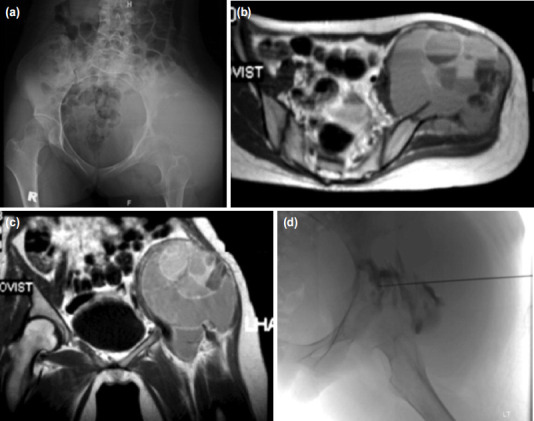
(a) Plain radiograph of pelvis showing a lytic lesion at left iliac region, without involvement of the left acetabulum and a narrow zone of transition with soft tissue shadow extension. (b) MRI pelvic axial view and (c) coronal view revelved a well demarcated lesion at left iliac with multiple fluid-fluid levels. Gluteal and iliopsoas muscle were pushed by expansile ilium and no evidence of infiltration. (d) Sclerosing injection to the pelvic.
